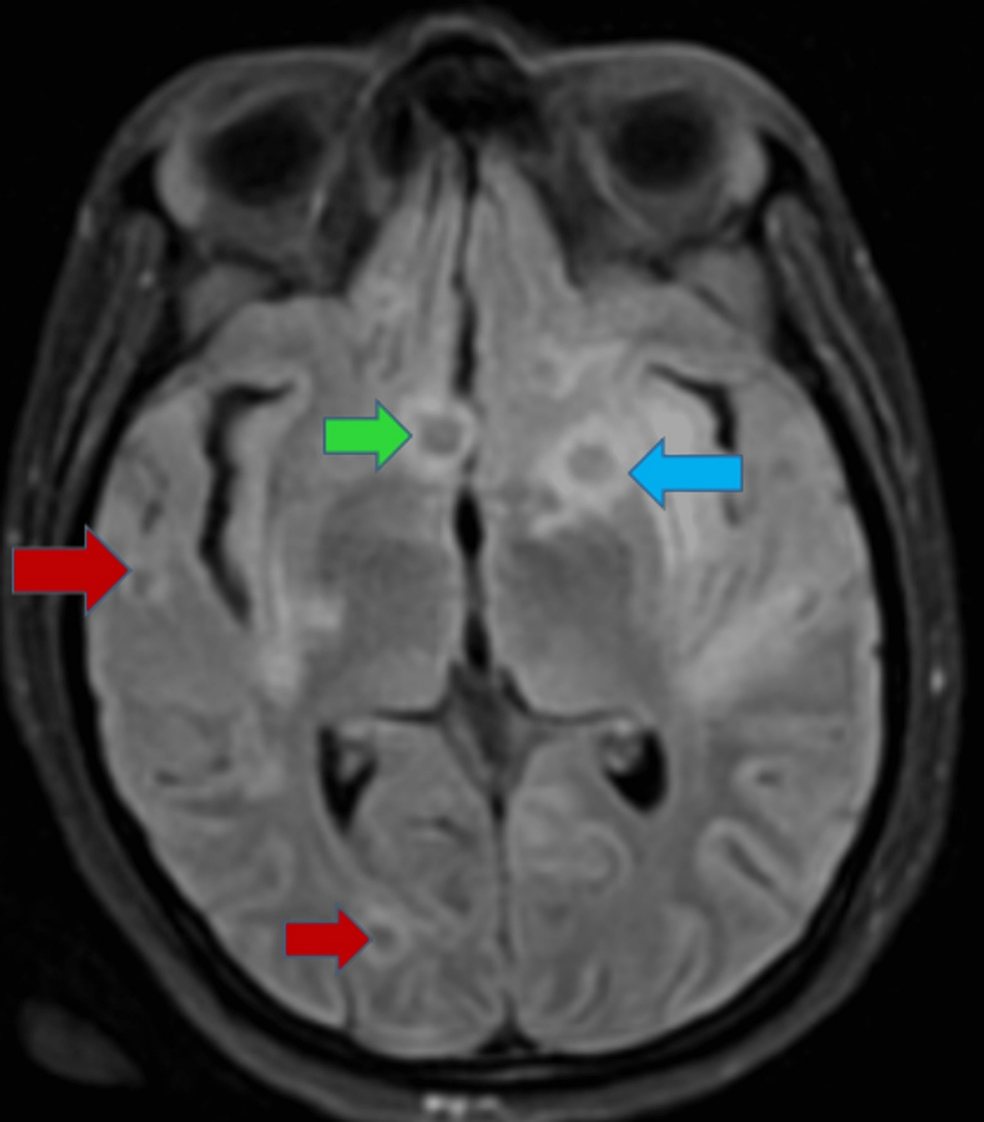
FLAIR MRI of the brain in axial projection Hypointense ring-enhancing lesions are represented by arrows at the gray-white matter junction (red), left capsuloganglionic region (blue), and medial temporal lobe (green). FLAIR: fluid-attenuated inversion recovery.
